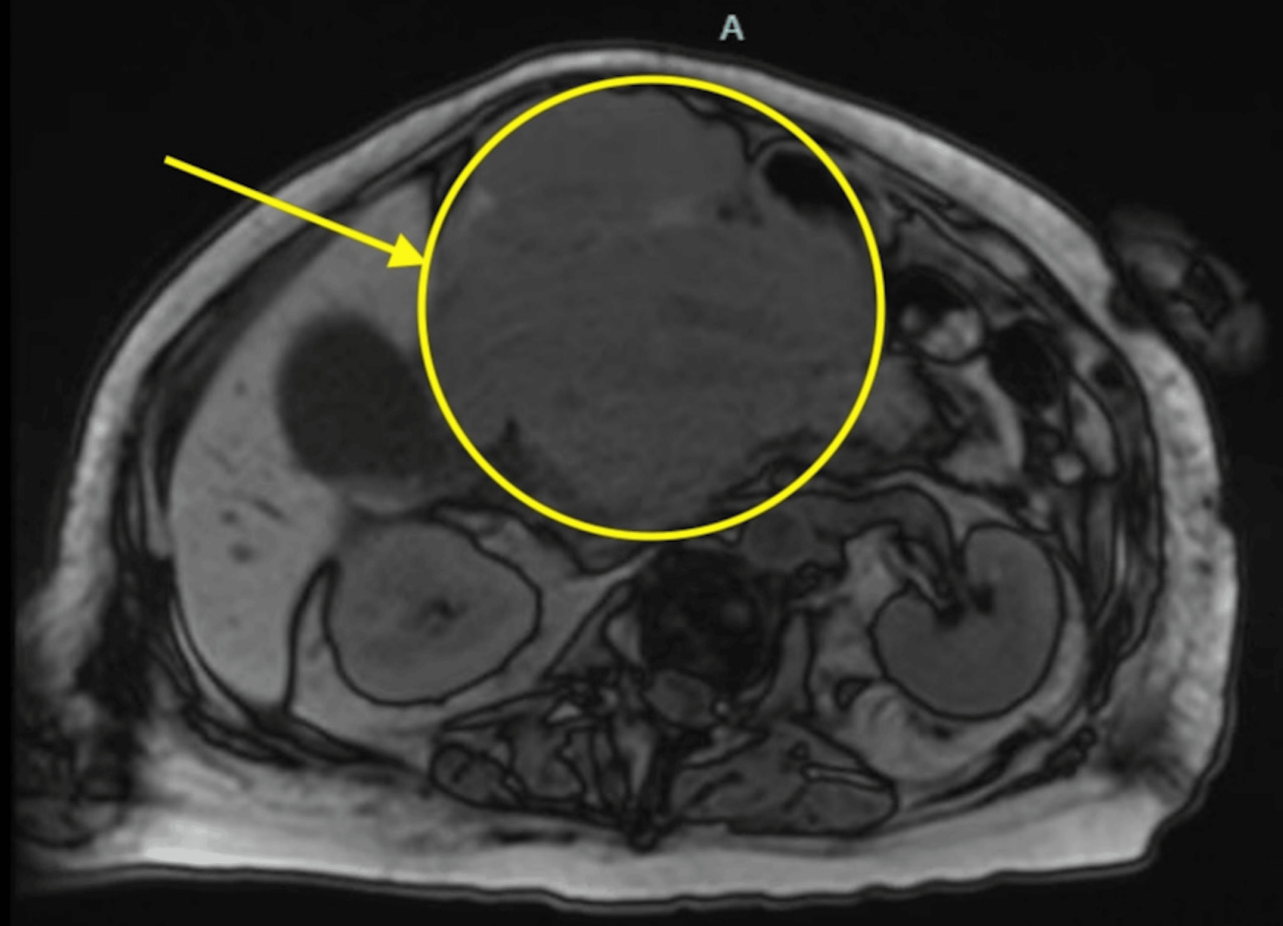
Axial view of magnetic resonance cholangiopancreatography. The image displays an axial cross-section of the patient’s magnetic resonance cholangiopancreatography scan taken during hospital admission to better visualize the origin of the abdominal mass. The yellow arrow and circle highlight the large abdominal mass, as seen on initial CT scan in Figures 1, 2.
